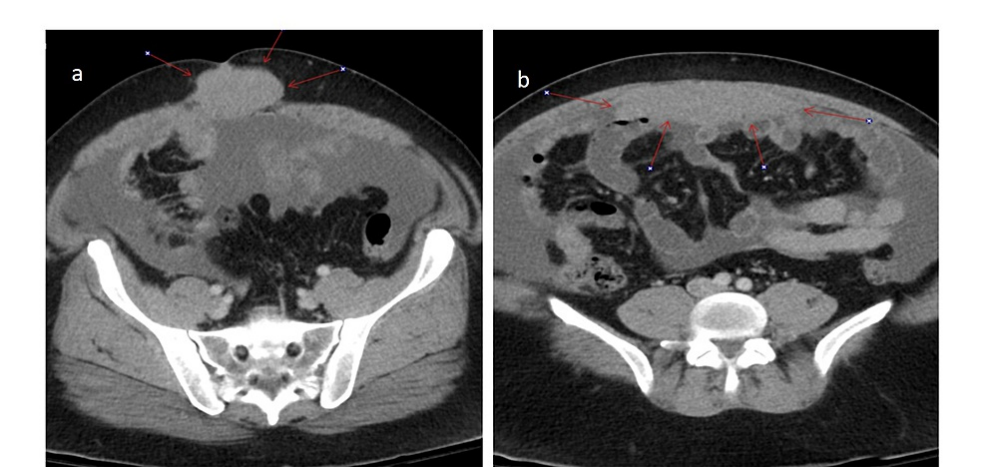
(a) Axial section of CT abdomen/pelvis with contrast showing mass in the umbilical region (arrows); (b) Axial section of CT abdomen/pelvis with contrast showing showed diffuse thickening and soft tissue attenuation of the omentum along with moderate volume ascites concerning for omental carcinomatosis (arrows).
